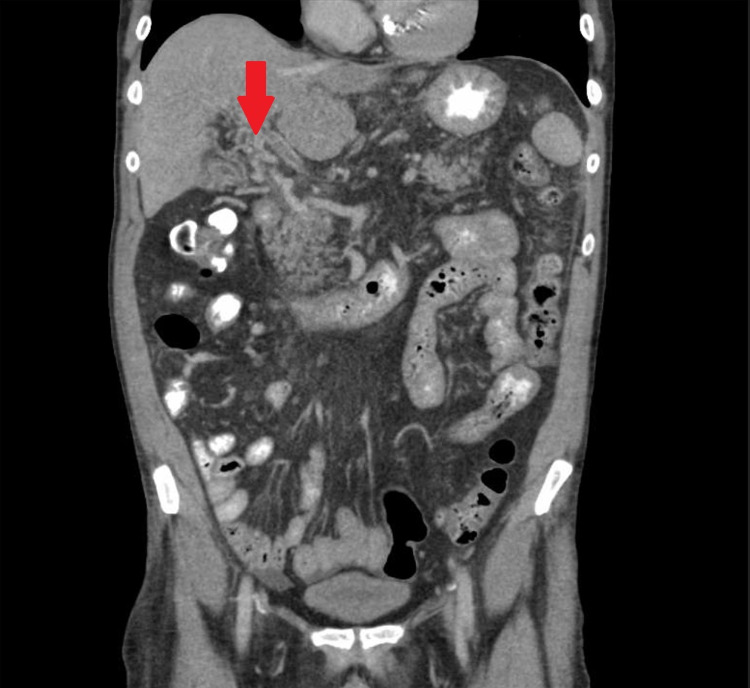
CT scan of the abdomen with IV contrast (coronal view). Arrowhead demonstrates the cavernous transformation of the portal vein and thrombus within the portal vein.
